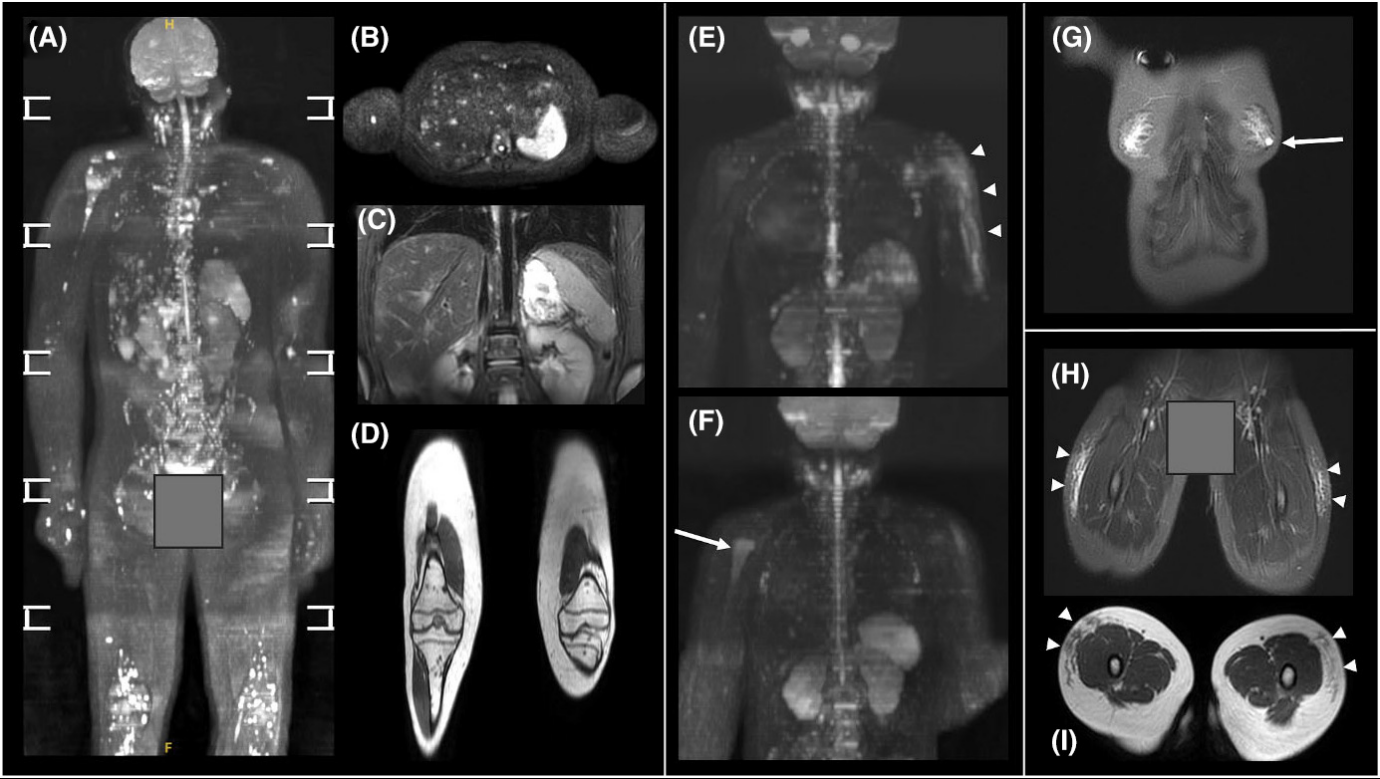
Examples of positive scan findings from four participants: (A) 3D‐rotational MIP b900 DWI image demonstrating multifocal granulomatous disease involving bones, liver and skin; (B) Axial b900 image from the same participant showing the multiple hyperintense liver lesions, as well as lesions in the right humeral shaft and vertebral body; (C) coronal STIR image from the same participant showing multiple hyperintense liver and vertebral body lesons; (D) coronal T_1_‐weighted mDIXON image through the legs of the same participant showing multiple femoral and tibial bone marrow hypontense foci; (E) 3D‐rotational MIP b50 image showing subcutaneous oedema left upper arm (arrow heads); (F) b900 DWI image from the same participant showing high signal right upper humerus (arrow); (G) coronal STIR image showing left sub‐areolar cyst (arrow); (H) coronal STIR and (I) axial T_1_ from a participant with bilateral anterolateral thigh subcutaneous oedema.
